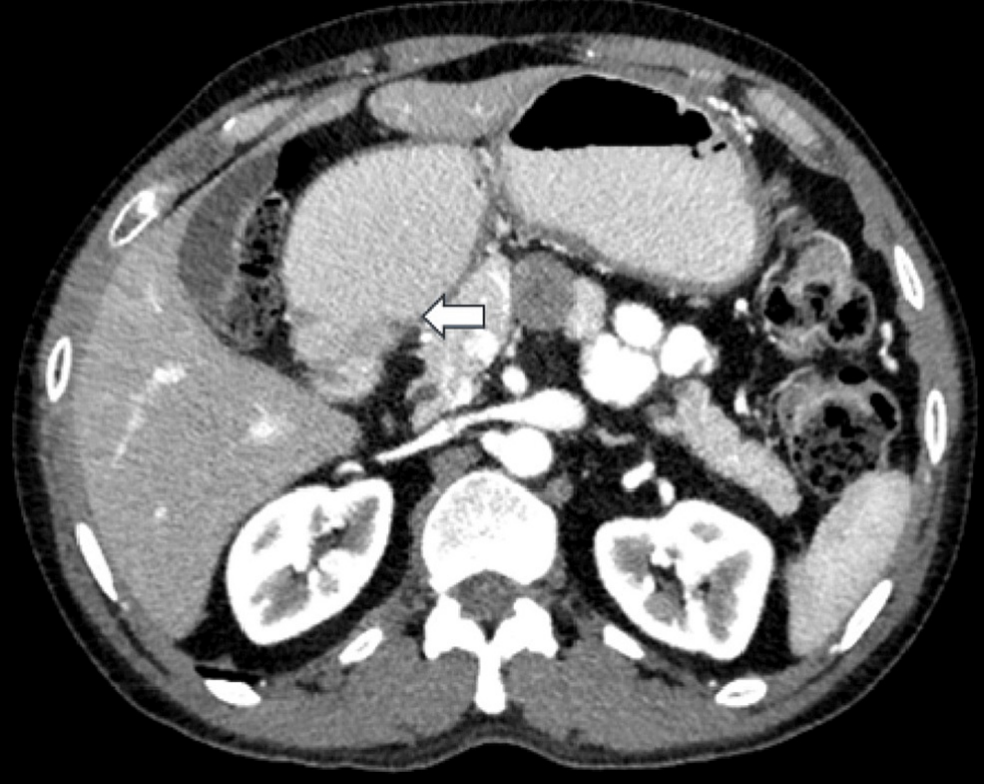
Repeat CT scan of the abdomen with contrast (axial view) showing regression of circumferential and asymmetric wall thickening at the pyloric region (arrow) and regression of dilatation of the stomach
